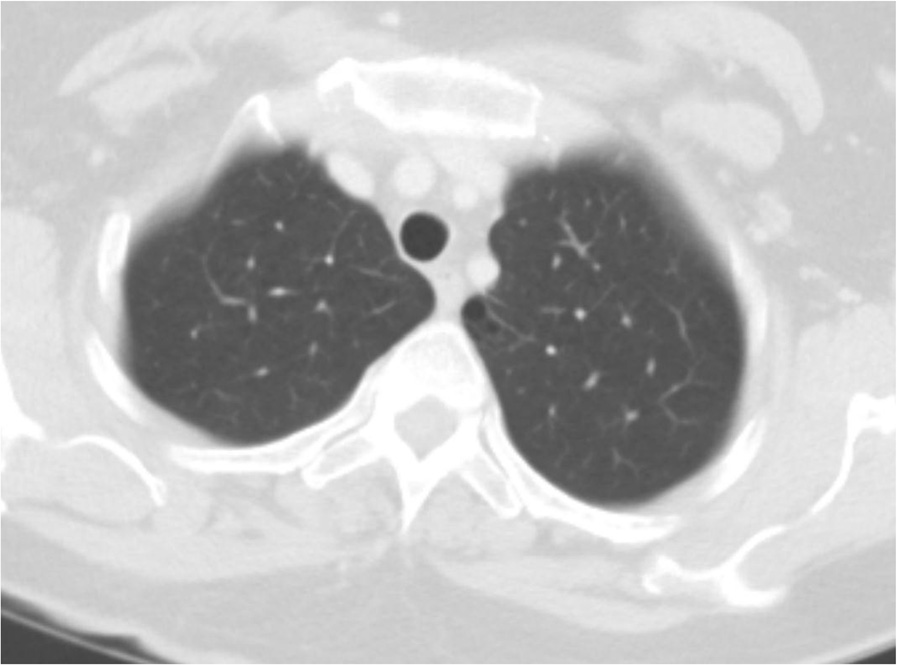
Computed tomographic scan of the chest taken 9 months prior to admission. This image shows a small cluster of emphysema at the medial left upper lobe, but otherwise the lung parenchyma is normal.
